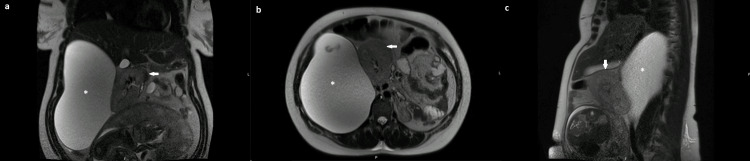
Abdominal MRI: T2 sequence: very large retroperitoneal lesion in the right flank (*), with homogeneous hypersignal pattern and regular and well-defined contours, pushing the bowel loops and the right kidney anteriorly, molding to the posterior surface of the right kidney. (a) Coronal section. (b) Axial section. (c) Right parasagittal section. The arrow points to the right kidney with medial and anterior displacement. MRI: magnetic resonance imaging
